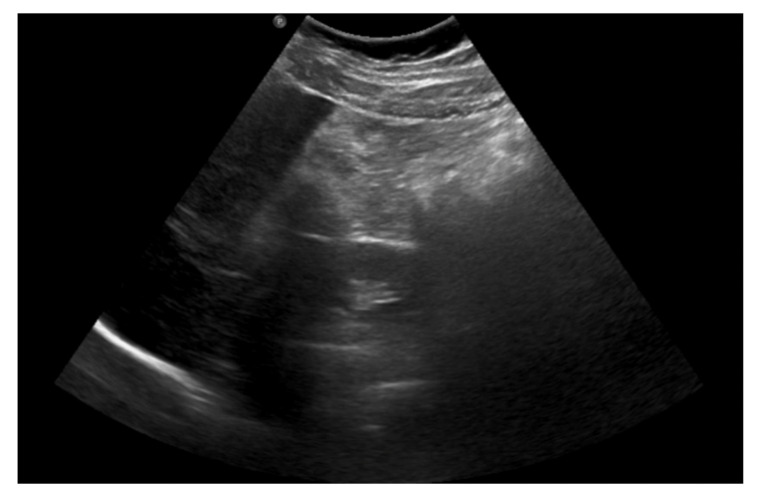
The abdominal ultrasound on admission: mesenteric adipose tissue with increased echogenicity and free fluid.
